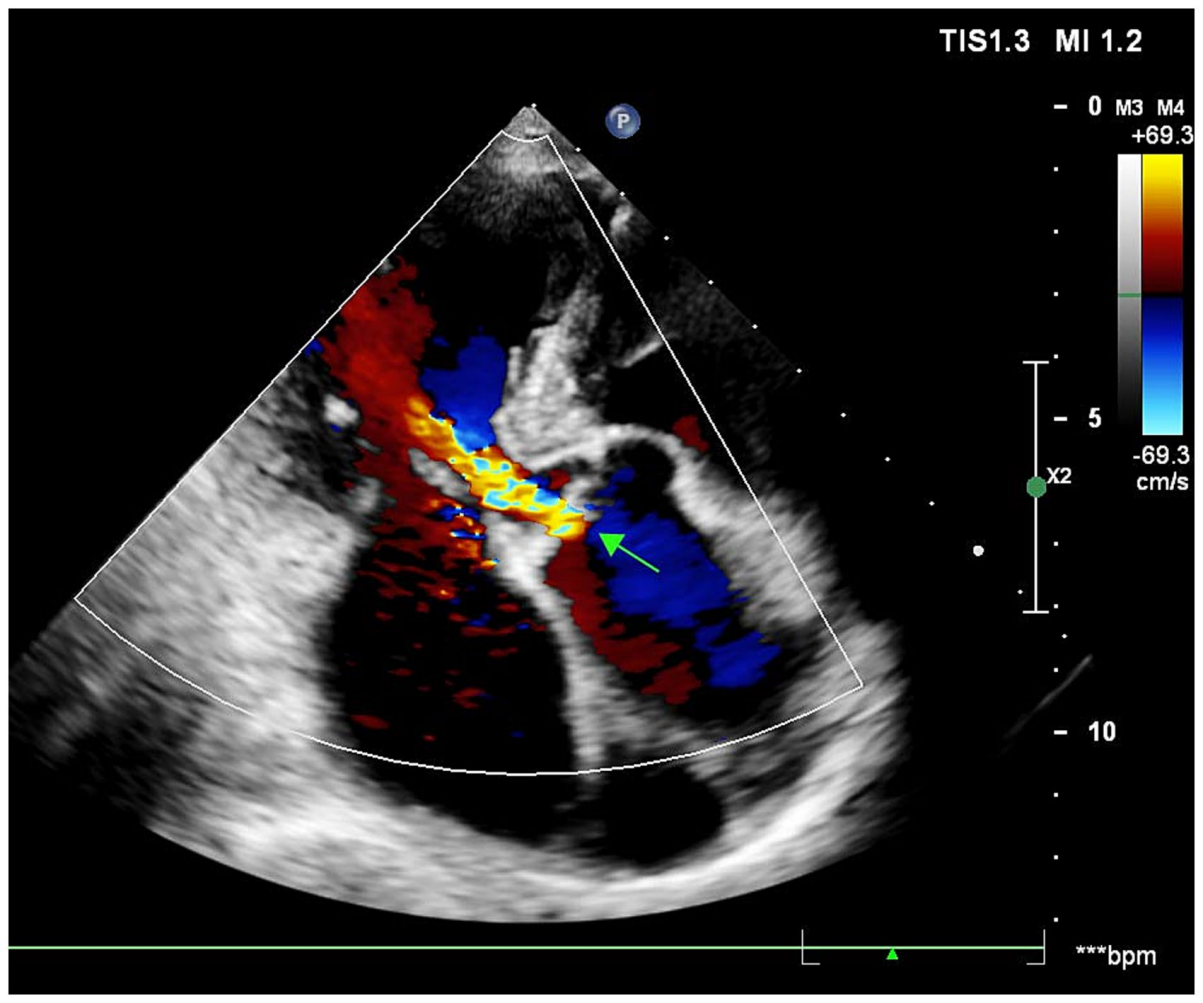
Echocardiographic features of aortic valvulopathy.
